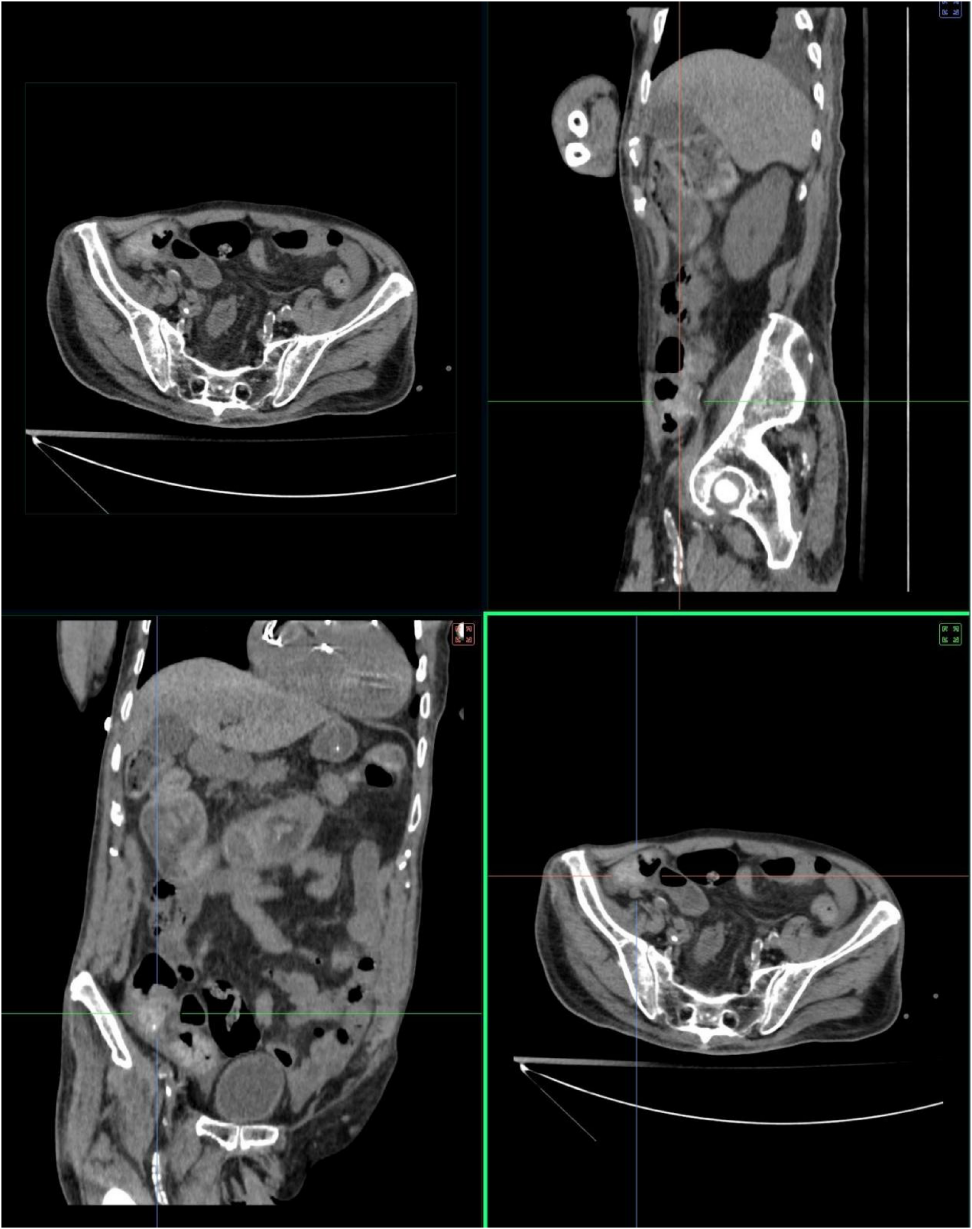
One week after the acupuncture intervention, sagittal CT of the abdomen demonstrating resolution of intestinal obstruction and significant reduction in abdominal distension.
